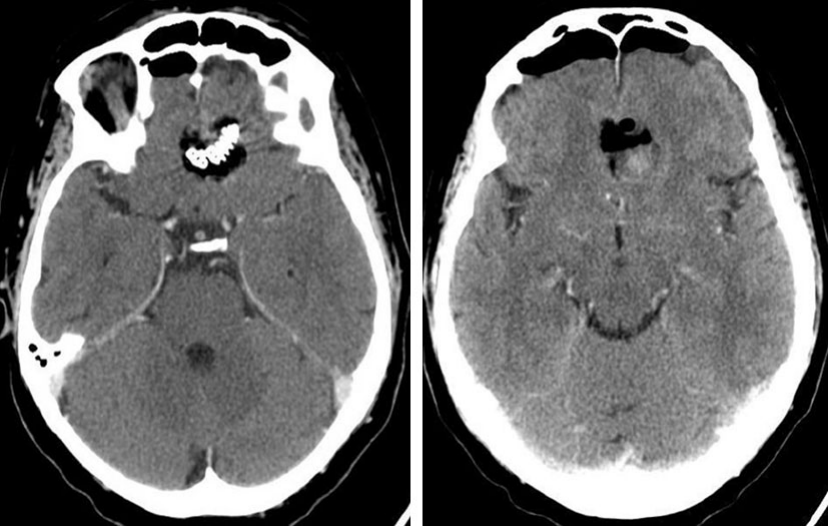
Case number 1. Follow up CT scan. Skull base reconstructed with a titanium mesh
